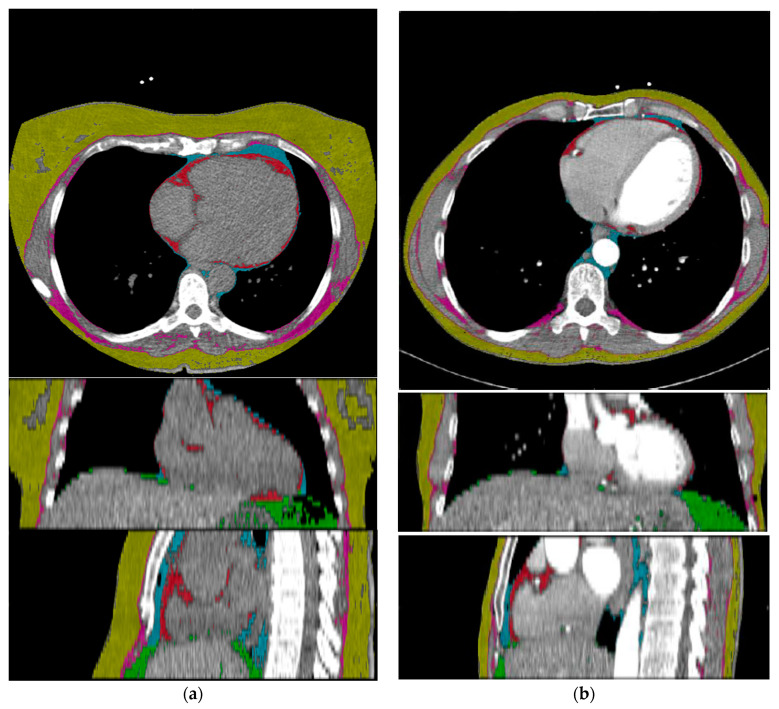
Exemplary adipose tissue predictions of a non-contrast-enhanced Chest CT (a) and a contrast-enhanced Chest CT scan (b). Description of labels and colors: yellow: subcutaneous adipose tissue, pink: intermuscular adipose tissue, cyan: PAT, red: EAT, green: visceral adipose tissue.
